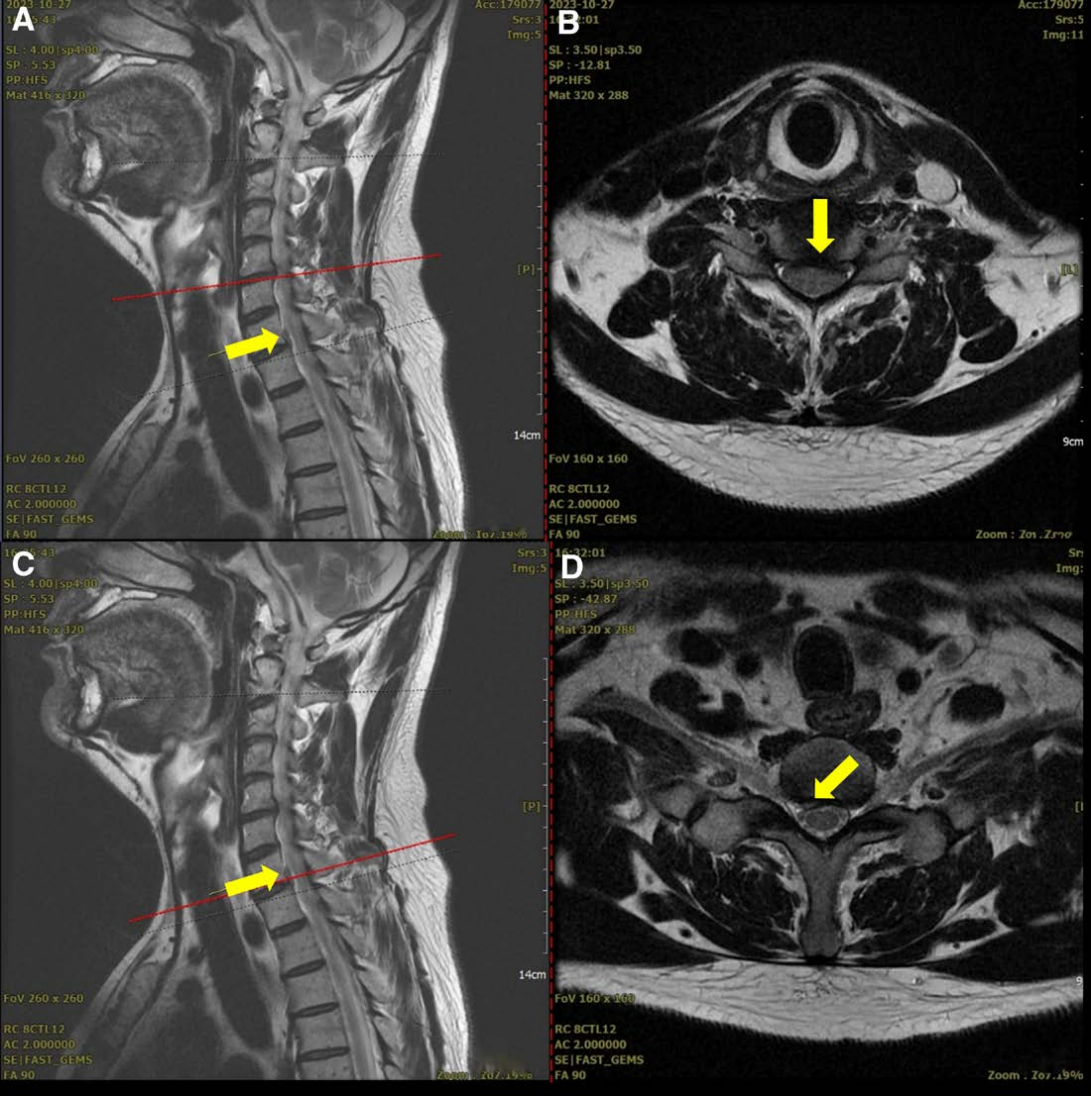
C5/6 magnetic resonance imaging on October 27, 2023 (A, B) and C7/T1 magnetic resonance imaging on October 27, 2023 (C, D).
